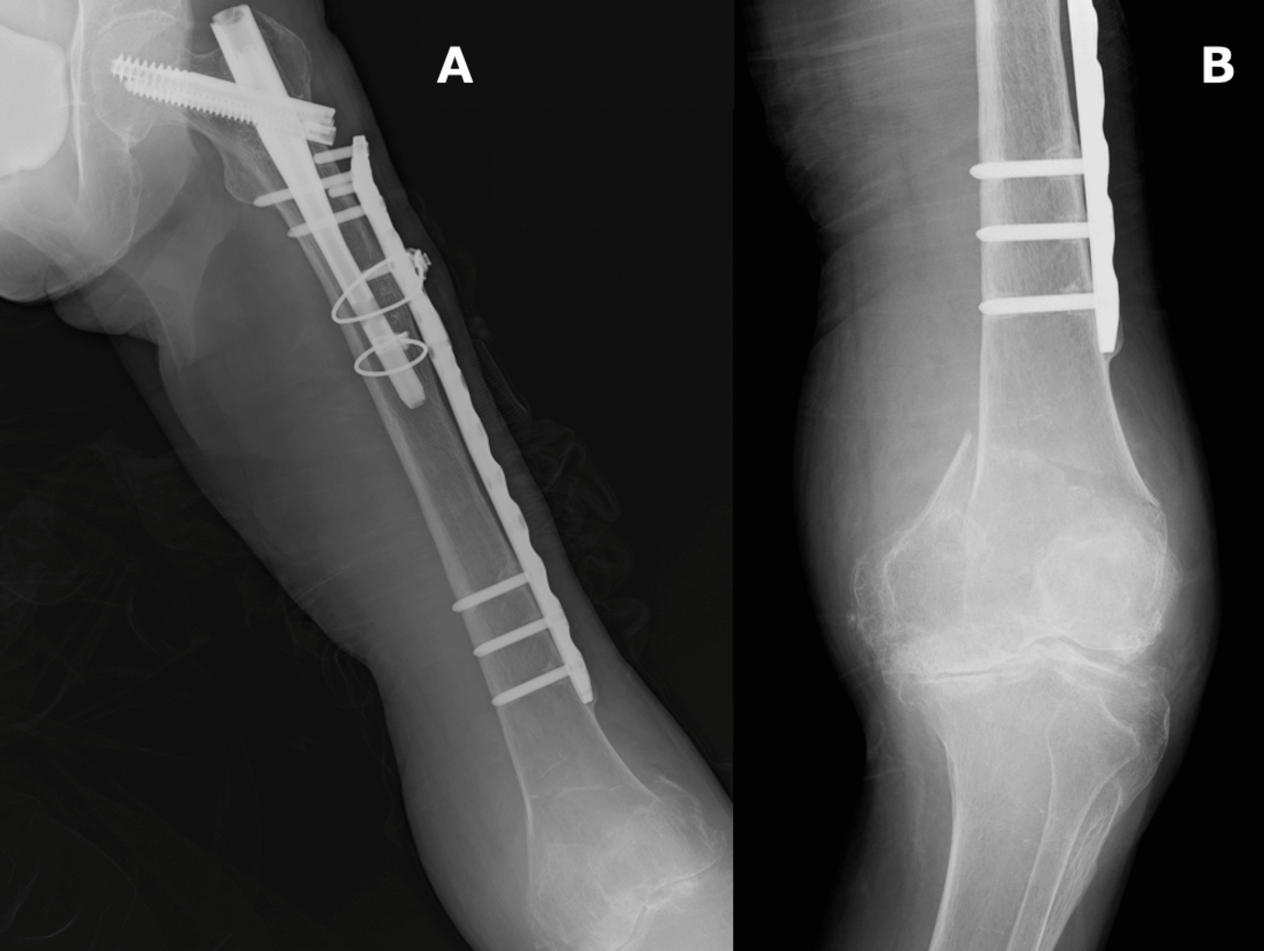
Radiographic examinations A: preoperative full-length anteroposterior radiograph of the femur; B: preoperative anteroposterior radiograph of the knee
